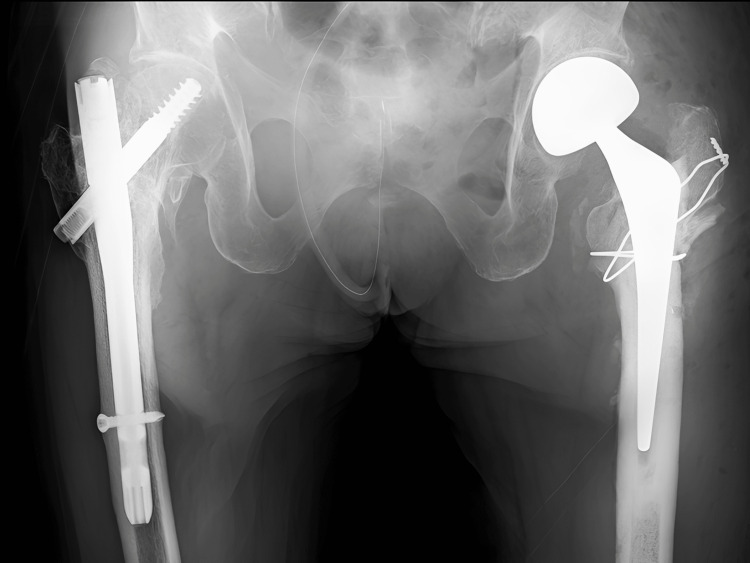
X-ray images after reoperation (cemented bipolar hemiarthroplasty). The unstable greater trochanter was stabilized by wiring.
